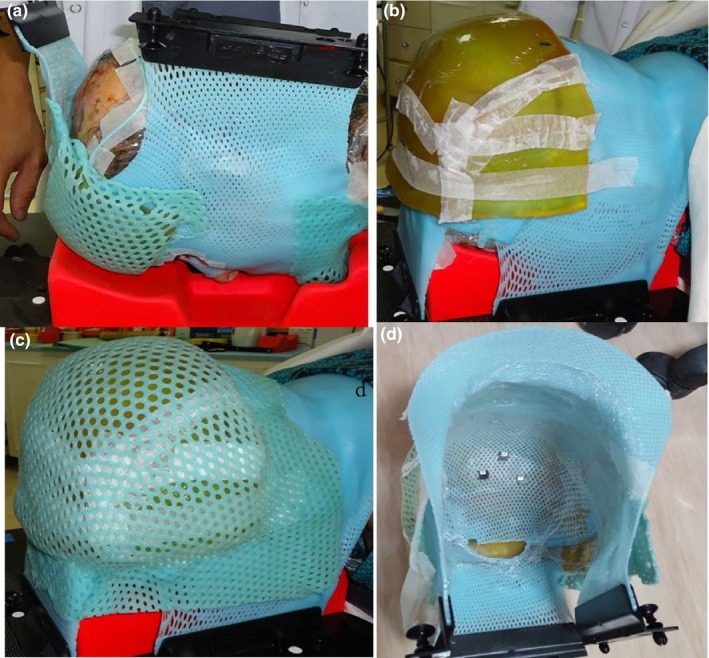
Steps for the posterior DSB device, (a) Picture of the posterior inner shell, (b) Bolus was in placed over the posterior inner shell, (c) Outer shell was placed over the bolus, (d) Picture of the final posterior DSB with three OSLDs in placed.A posterior scout image was obtained to verify the patient's alignment.The anterior mask was fixed to the prone headrest (a glue gun was used).A three‐point shell was placed in a hot water bath until translucent. The shell was then patted dry and stretched over the patient's posterior head and neck area. A good molding of the shell over the neck and skull area was ensured.When the posterior shell was set and completely dried, the bolus was added. The thickness and area of the bolus were determined by the radiation oncologist and verified by the medical physicist. During application, a better attachment to the shell was achieved by removing the skin of the bolus and placing its sticky side facing the shell. We ensured that there were no gaps or overlap resulting from the irregular shape of the area to be covered.A thermoplastic sheet was placed in a hot water bath. Next, the sheet was positioned over the bolus and attached to the posterior shell (the bolus was permanently sandwiched between the shells). As advised by the medical physicist and the radiation oncologist, the therapist performed a CT scan of the patient in the treatment position (see Section 2.D).
